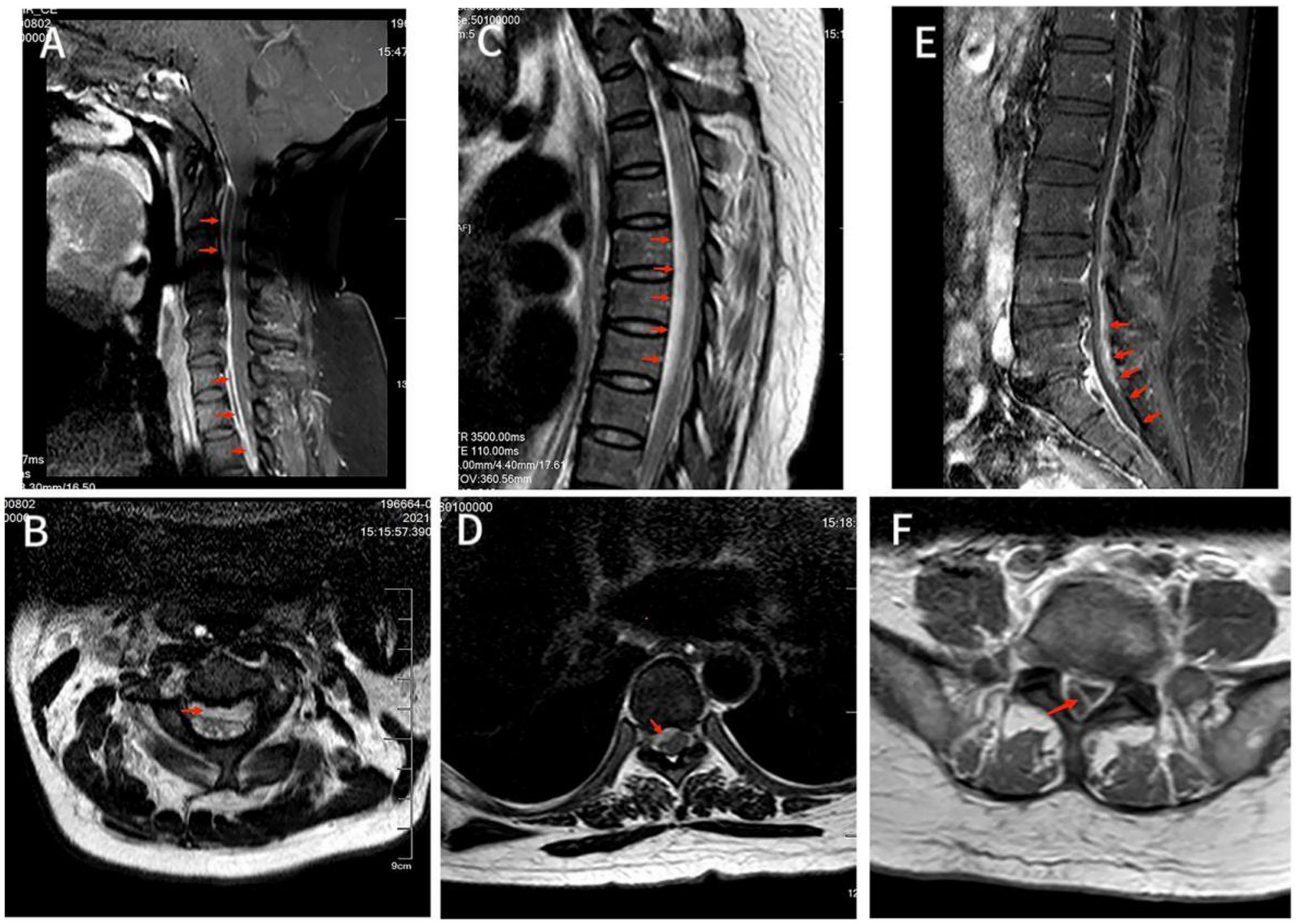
(A) MRI T2-weighted image showed hyperintensity of the lesion on the ventral side of the spinal cord in the C2-C6 spinal canal. Also saw C5/6 intervertebral disc herniation and the corresponding dura and spinal cord were compressed. (B) Axial T2-weighted image showed an annular high signal lesion in the anterior part of the spinal cord within the spinal canal of C2, and spinal cord compression at the C2. (C) T2-weighted image showed hyperintensity of lesions ventral to the spinal cord in the T2-T8 spinal canal, with corresponding levels of spinal cord compression degeneration. (D) Axial T2-weighted image showed annular high signal lesion in the right anterior part of the spinal cord within the spinal canal of T7 accompanied by nerve root and spinal cord compression. (E) T1-enhanced image showed disc bulging in L3/4, L4/5 and L5/S1 with annular enhancement of the lesion ventral to the spinal cord in the L4-S1 spinal canal with spinal cord compression at the corresponding level, but no significant internal enhancement. (F) Axial T1-enhanced image showed a large range of irregular non-enhanced areas in the soft tissue of the right posterior side of the at the L5 level, and a ring-enhancing lesion in the right posterior part of the intradural sac with no obvious internal enhancement.
